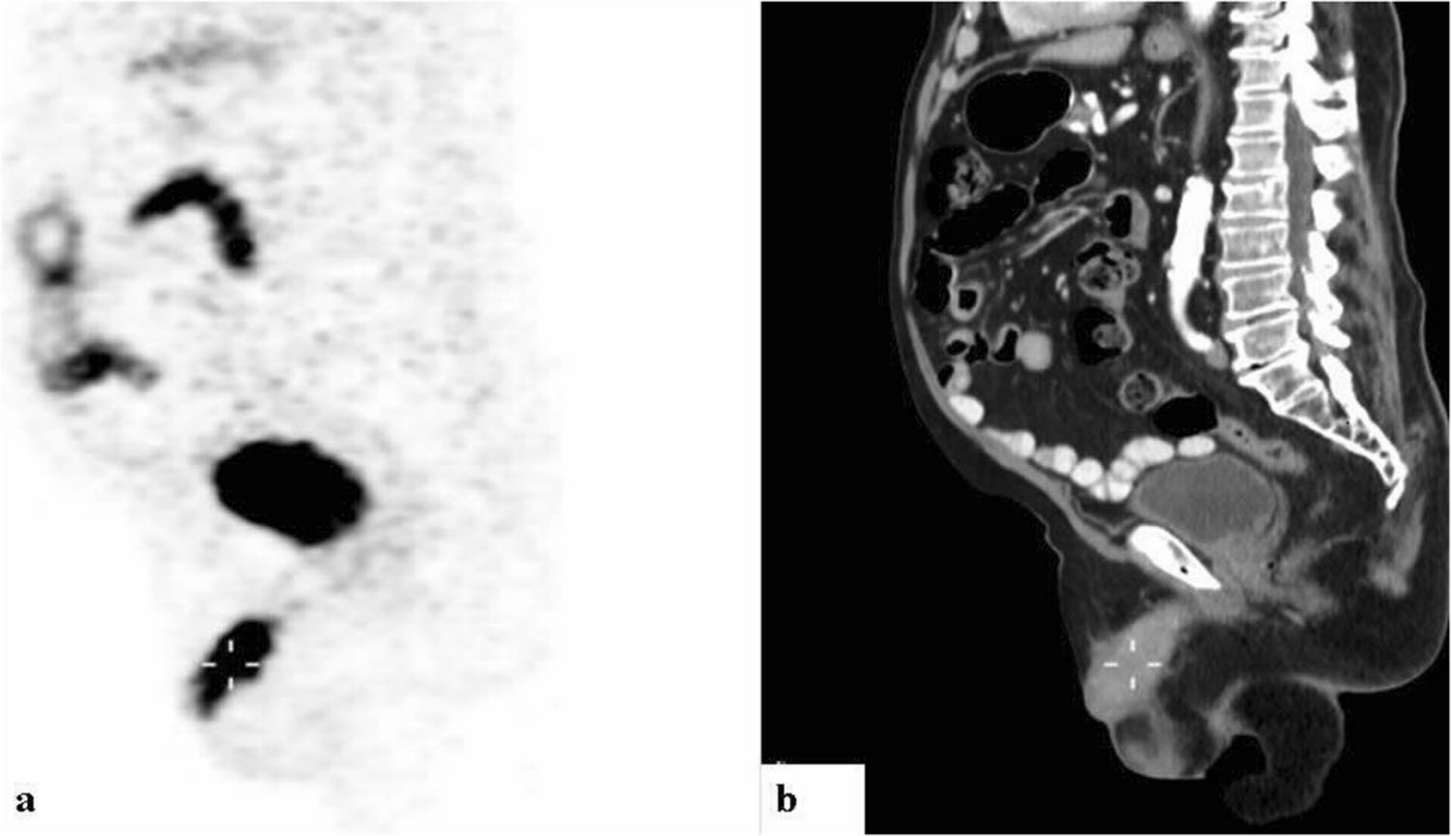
Ga-68 PSMA-PET (a) and CT (b) sagittal slices demonstrating diffusely increased uptake along the penis. A 71-year-old man with metastatic prostate cancer of neuroendocrine differentiation, in the absence of prior prostatectomy, with a Gleason’s score of 7 and a PSA of 3 (patient #5, Table 1).
